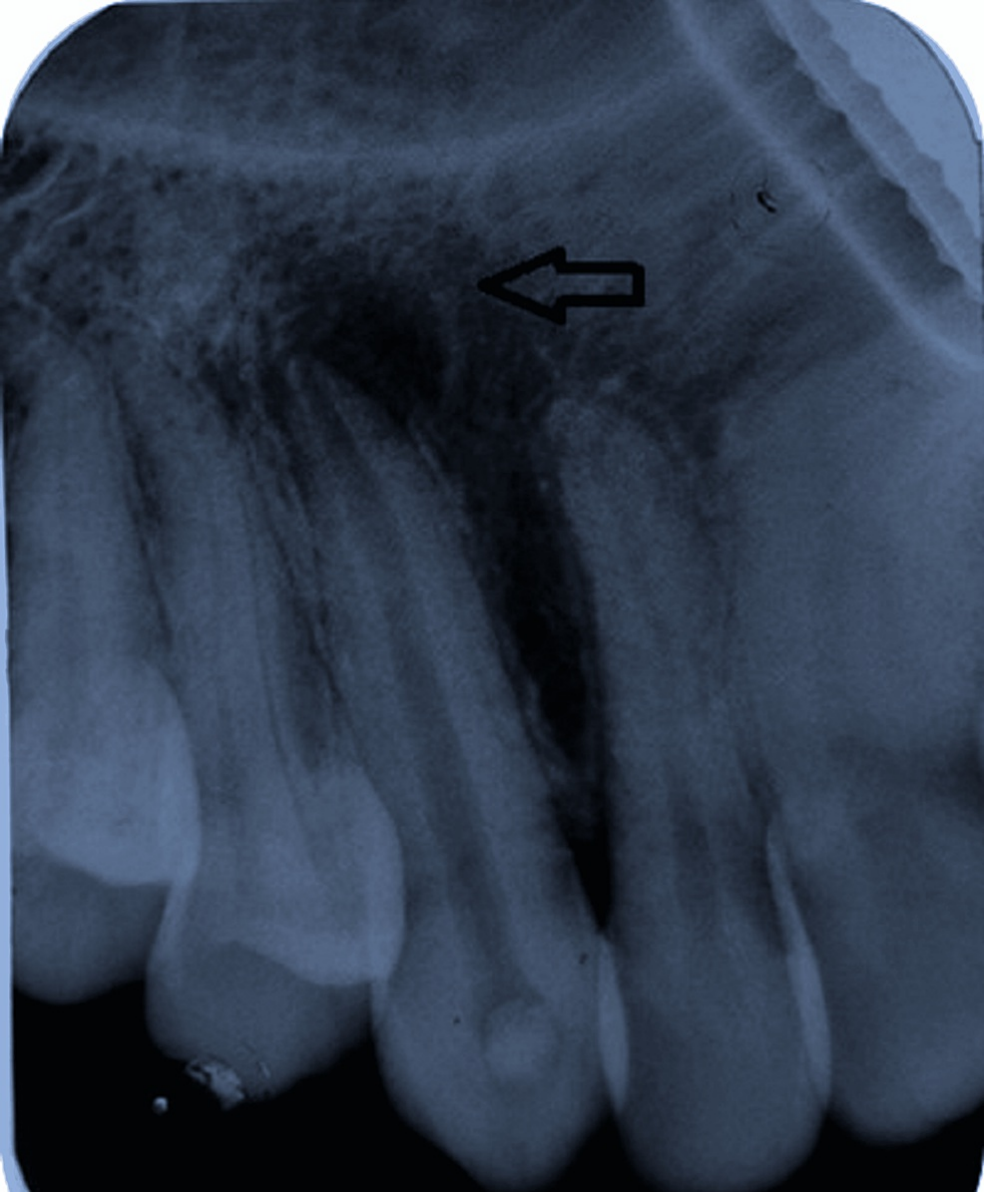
Periapical radiograph recorded at the recall period of 6 months to visualize the status of the periapical region of tooth number 13.
